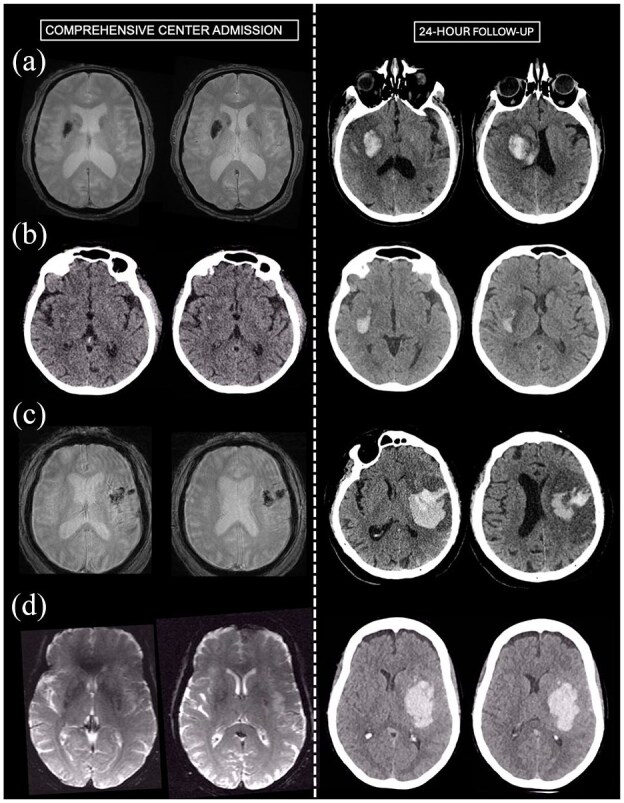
Representative cases with inter-hospital hemorrhagic transformation and subsequent expansion. (a) Patient admitted in the PSC with NIHSS of 22 and M1 occlusion. IVT was administered. Brain MRI on CSC admission 3.5 h later (NIHSS 20) shows an M1 recanalization (distal middle cerebral artery branch occlusion, rAOL 2b recanalization) and a deep HI-2 HT on T2*. EVT was not performed. 24-h follow-up CT shows HT expansion (from 6 to 15 cc). (b) Patient admitted in the PSC with NIHSS of 12 and M1 occlusion. IVT was administered. CT on CSC admission 2.5 h later (NIHSS 8) shows an M1 recanalization (distal middle cerebral artery branch occlusion, rAOL 2b recanalization) and a deep HI-1 HT. EVT was not performed. 24-h follow-up CT shows HT expansion (from <1 to 8 cc). (c) Patient admitted in the PSC with NIHSS of 16 and proximal M2 occlusion. IVT was administered. Brain MRI on CSC admission 3.5 h later (NIHSS 17) shows a stable M2 occlusion and a PH-1 HT on T2*. EVT was not performed. 24-h follow-up CT shows HT expansion (from 12 to 57 cc). (d) Patient admitted in the PSC with NIHSS of 17 and M1 occlusion. IVT was not administered. Brain MRI on CSC admission 3.7 h later (NIHSS 13) shows a slight M1 thrombus migration (rAOL 1) and a deep HI-2 HT on T2*. EVT was performed, with modified Thrombolysis In Cerebral Infarction score 2b achieved after one pass. 24-h follow-up CT shows HT expansion (from 1 to 77 cc). CSC: comprehensive stroke center; EVT: endovascular therapy; HI: hemorrhagic infarction; HT: hemorrhagic transformation; IVT: intravenous thrombolysis; PH: parenchymal hematoma; PSC: primary stroke center; M1 and M2: first, and second segment of the middle cerebral artery occlusion; rAOL: revised Arterial Occlusive Lesion scale.
